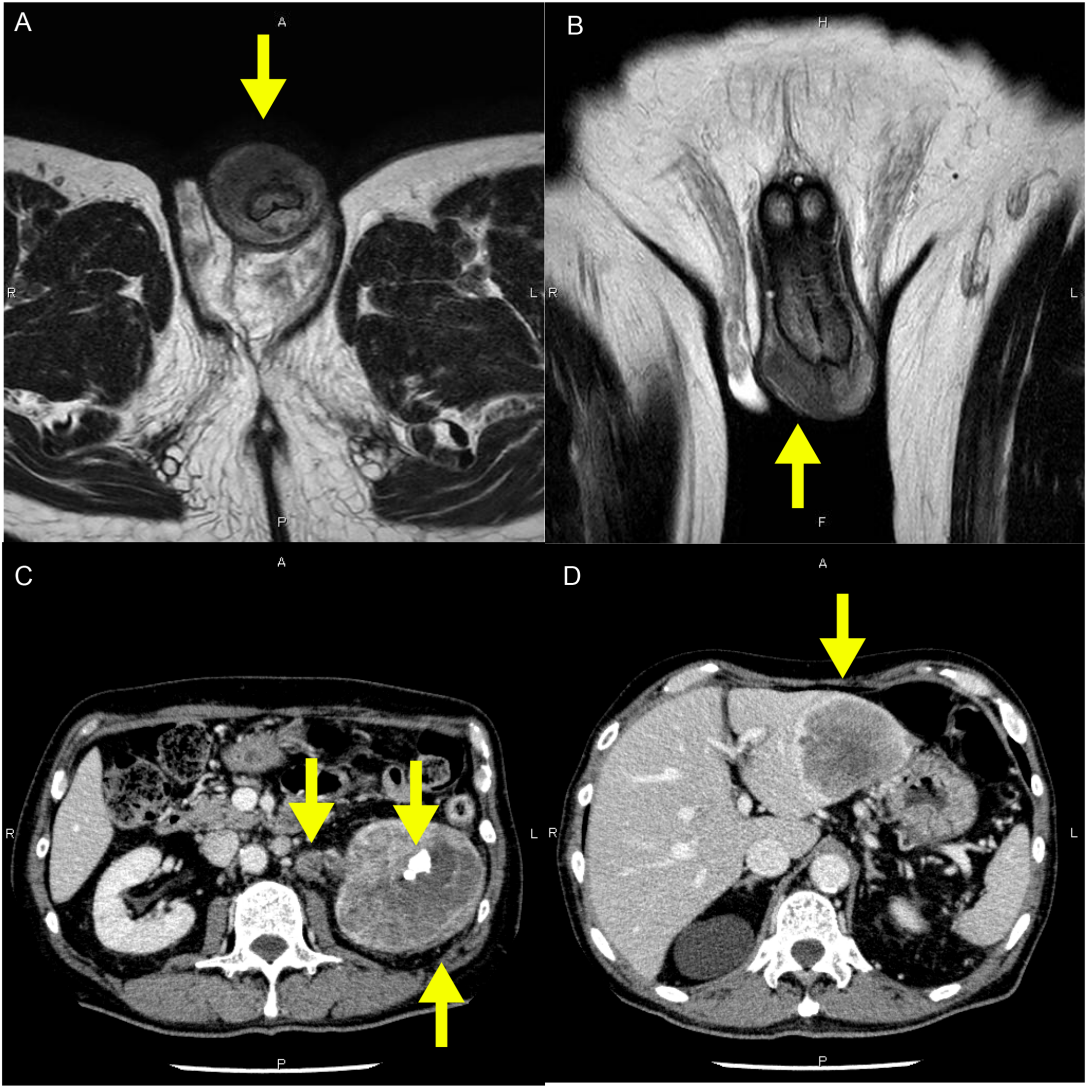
Magnetic resonance imaging (MRI) and contrast-enhanced computed tomography (CT) findings. (A, B) T2-weighted MRI of the penis in axial (A) and coronal (B) views showing a well-enhancing mass in the right glans (yellow arrows). (C, D) Contrast-enhanced abdominal CT demonstrating a large left renal pelvic mass with a renal calculus and hilar lymph node enlargement (C) and hepatic metastases (D). Yellow arrows indicate tumors, calculus, and metastatic lesions.
